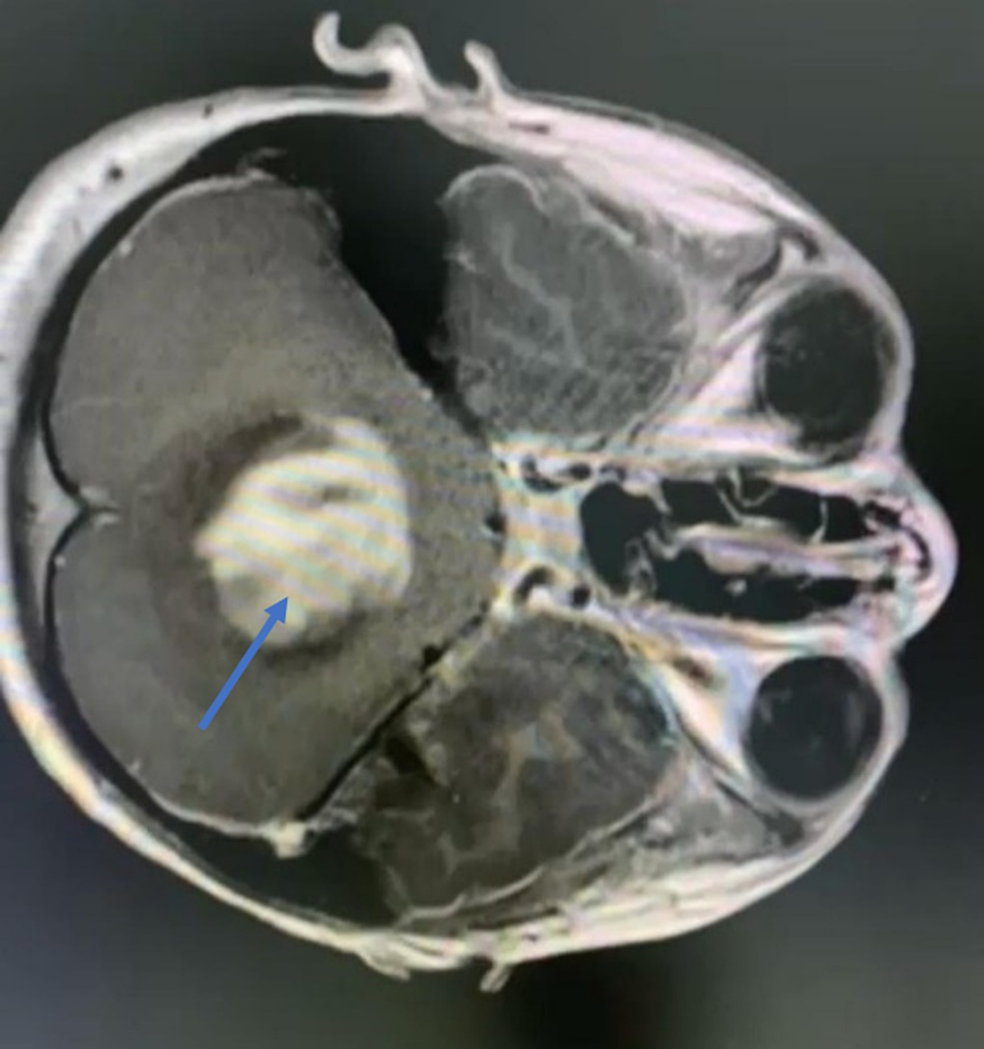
Preoperative image - MRI brain axial cut shows obstructive hydrocephalus
